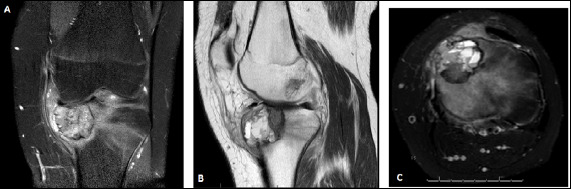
Magnetic resonance imaging of the left knee of a patient with chondroblastoma Caption A and B: Contrast-weighted magnetic resonance imaging on T2-weighted (A and C) and T1-weighted (B) sequences of the left knee of a 17-year-old boy with a history of severe pain for four months. The bone lesion lies in the proximal epiphysis of the tibia, measuring 4.0 cm, occupying 47.9% of the epiphysis and showing an eccentric shape. The coronal and sagittal sections show heterogeneous signals, perilesional spinal cord edema, synovitis in the adjacent joint, and a proximal tibial cartilage lesion. C: axial section showing rupture of the medial cortical fluid, which confirmed the secondary aneurysmal bone cyst in the anatomopathological analysis.
